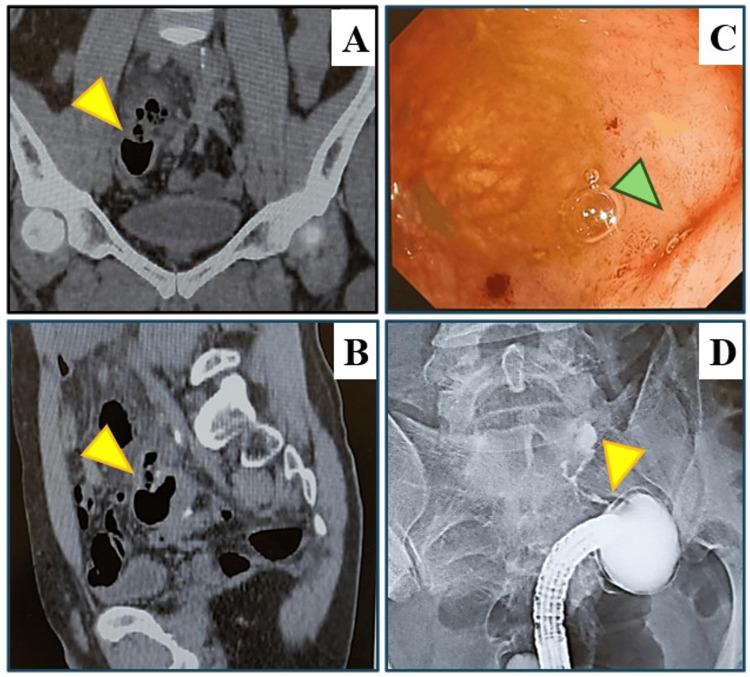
Initial imaging and fluoroscopy-assisted endoscopic findings Contrast-enhanced CT showed a localized pelvic abscess adjacent to the ileal pouch stump, or blind end, with small foci of free air, suggesting ileal pouch stump perforation and localized peritonitis (A (coronal view) and B (sagittal view)). Fluoroscopy-assisted pouchoscopy identified a small fistulous opening at the blind end with surrounding erythema (C). Contrast injection demonstrated localized extravasation into the abscess cavity (D). Yellow arrowheads indicate the suspected perforation site or contrast leakage, and the green arrowhead indicates the endoscopically identified fistulous opening. CT: computed tomography
